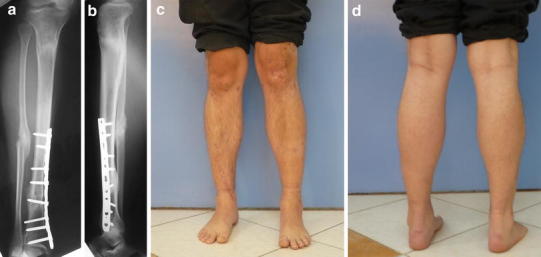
a–d follow-up radiographs and clinical photographs after consolidation of the nonunion with restoration of limb alignment
